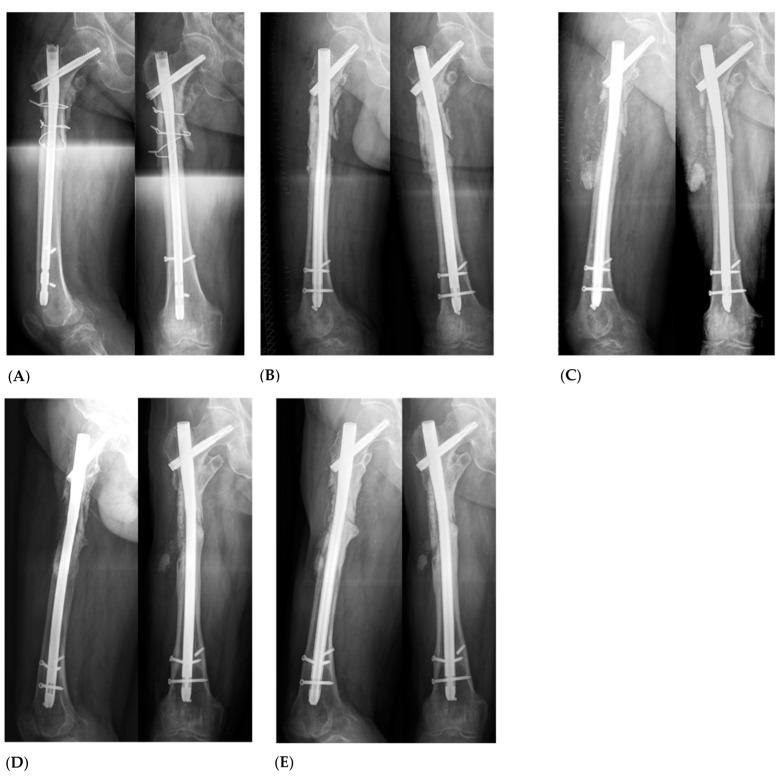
Case of a 55-year-old patient with atrophic nonunion following nail fixation of the proximal femur after subtrochanteric femur fracture (A). After performing Masquelet Step I with debridement of the atrophic bone, a significant bone defect exceeding 5 cm in size was observed (B). To address it, BaG was used as a bone substitute in combination with autologous bone using the RIA (Reamer Irrigator Aspirator) technique (C). At the 1-year follow-up (D), X-rays revealed complete bone consolidation, and ongoing bone remodeling was observed after 2 years (extended follow-up) (E).
